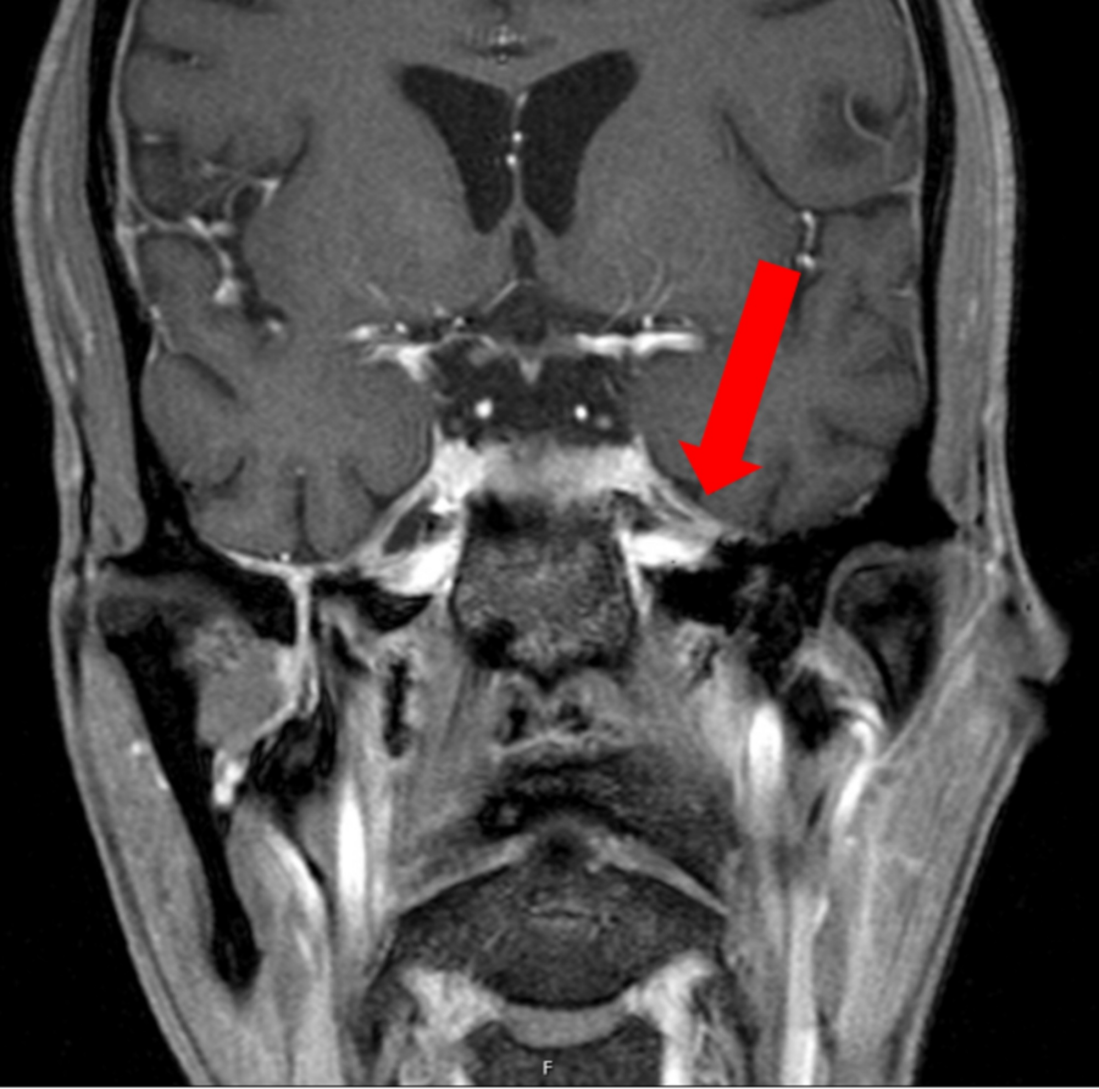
MRI of the brain with contrast The image shows focal meningeal thickening compressing the cistern of the left trigeminal ganglion (red arrow) MRI: magnetic resonance imaging
